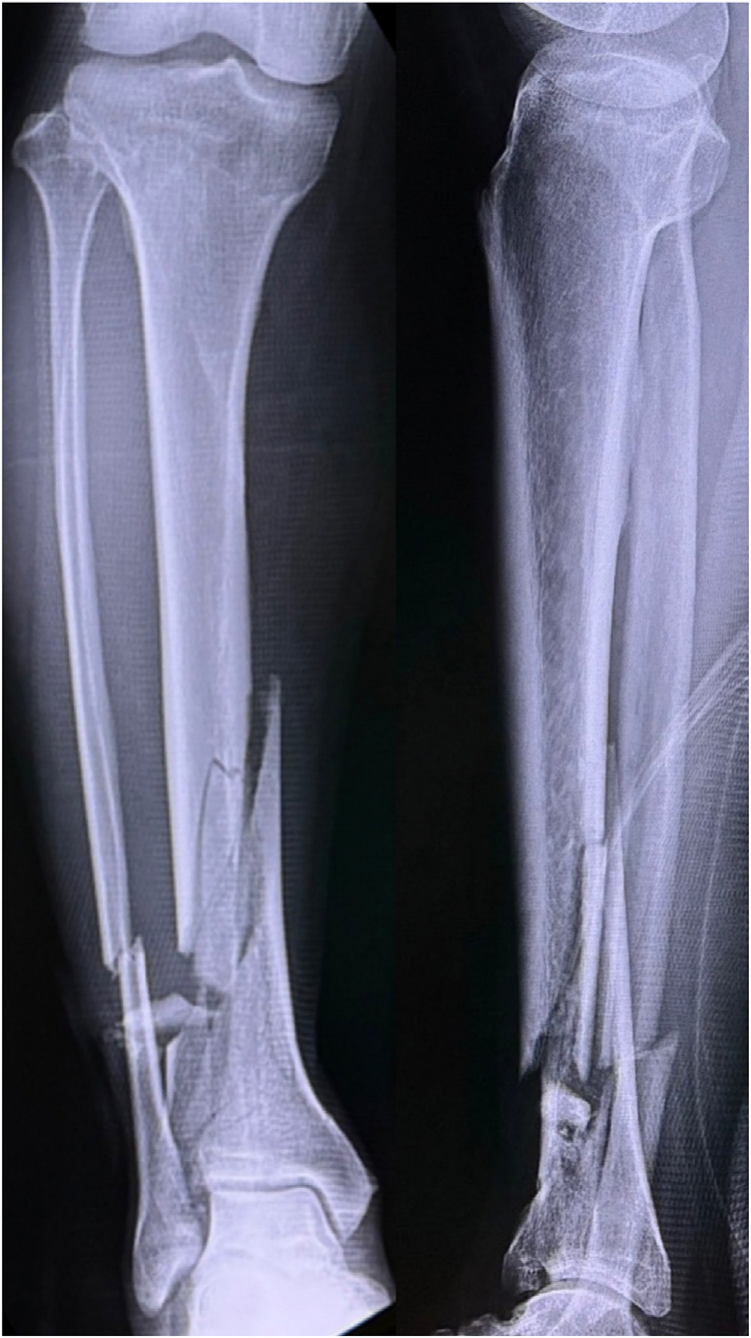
Plain radiograph at the time of injury.
